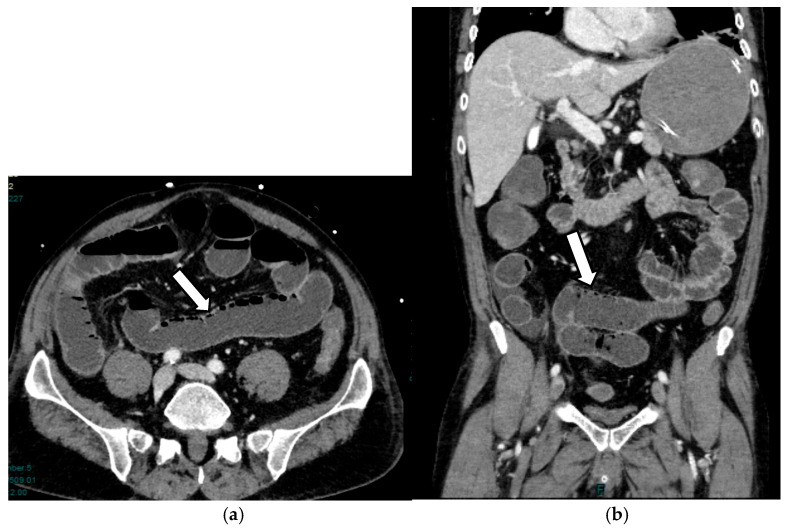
AAMI in a 56-year-old man: axial (a) and coronal (b) post-contrast CT images show dilated bowel loops, thinned bowel walls with decreased enhancement, and initial signs of pneumatosis (arrows).
